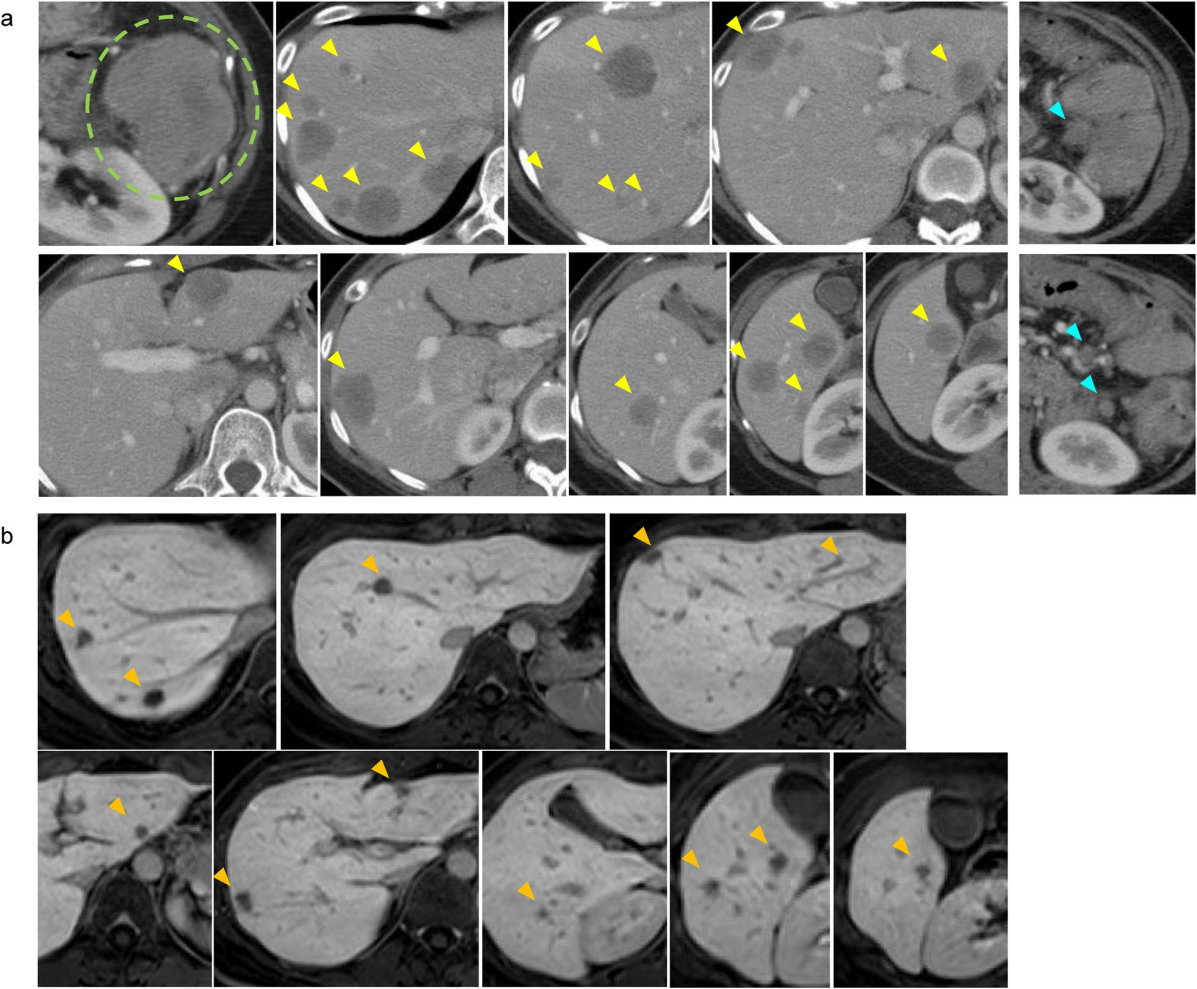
a Computed tomography at the initial examination showed thickened walls of the transverse colon at the splenic flexure (green circle), swollen lymph nodes in the mesocolon near the tumor (blue allows), and 19 multiple solid low-density masses in the liver (yellow allows). b Magnetic resonance imaging after chemotherapy showed shrinkage of all liver metastases in both lobes (orange arrows)
